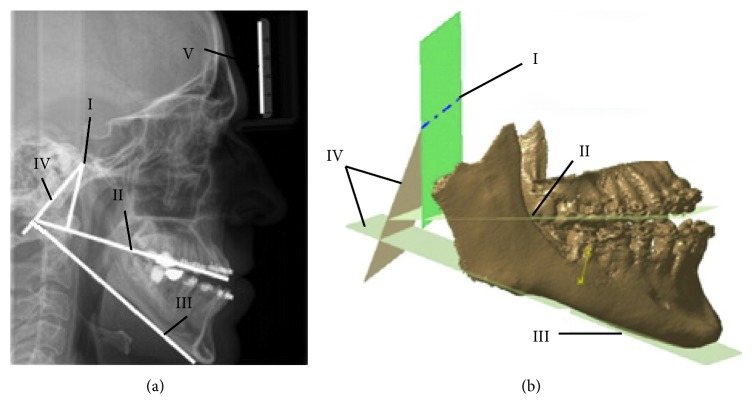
Assembly of personalized plate implant and bone 3D models: (a) X-ray image in LM plane—point of rotational axis (I), occlusion line (II), mandibular line (III), perpendicular lines (IV), etalon (V); (b) rotational axis (I), occlusion plane (II), mandibular plane (III), and perpendicular planes (IV).
